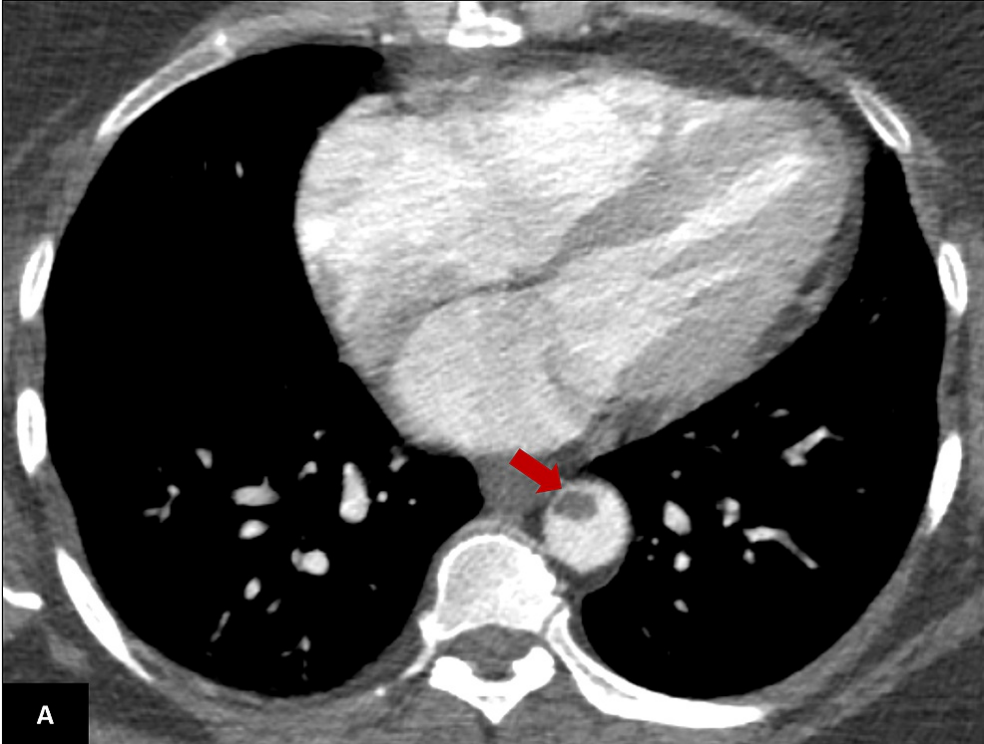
Axial contrast-enhanced CT scan of the lower thorax demonstrating a filling defect within the lower thoracic aorta representing a thrombus (red arrow).
